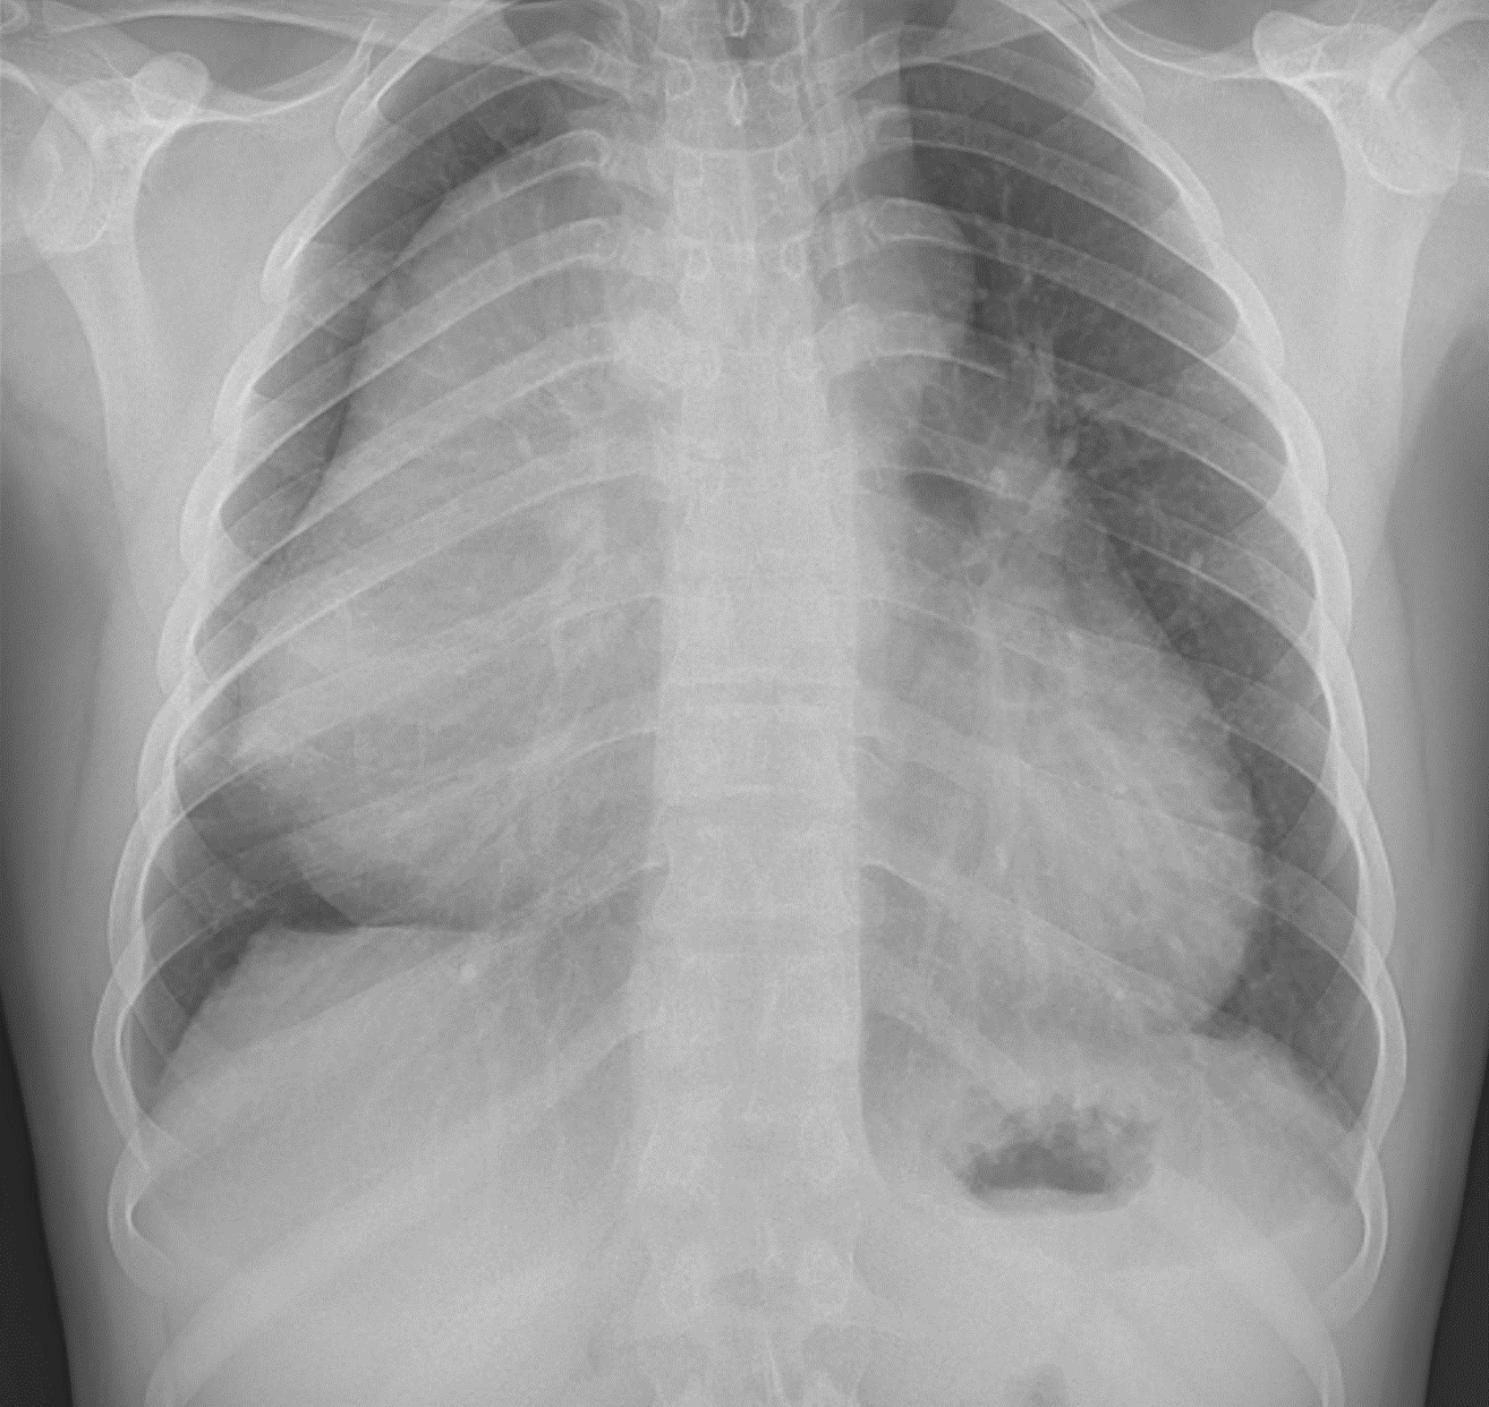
Chest x-ray showing a huge mass in the right mediastinum
